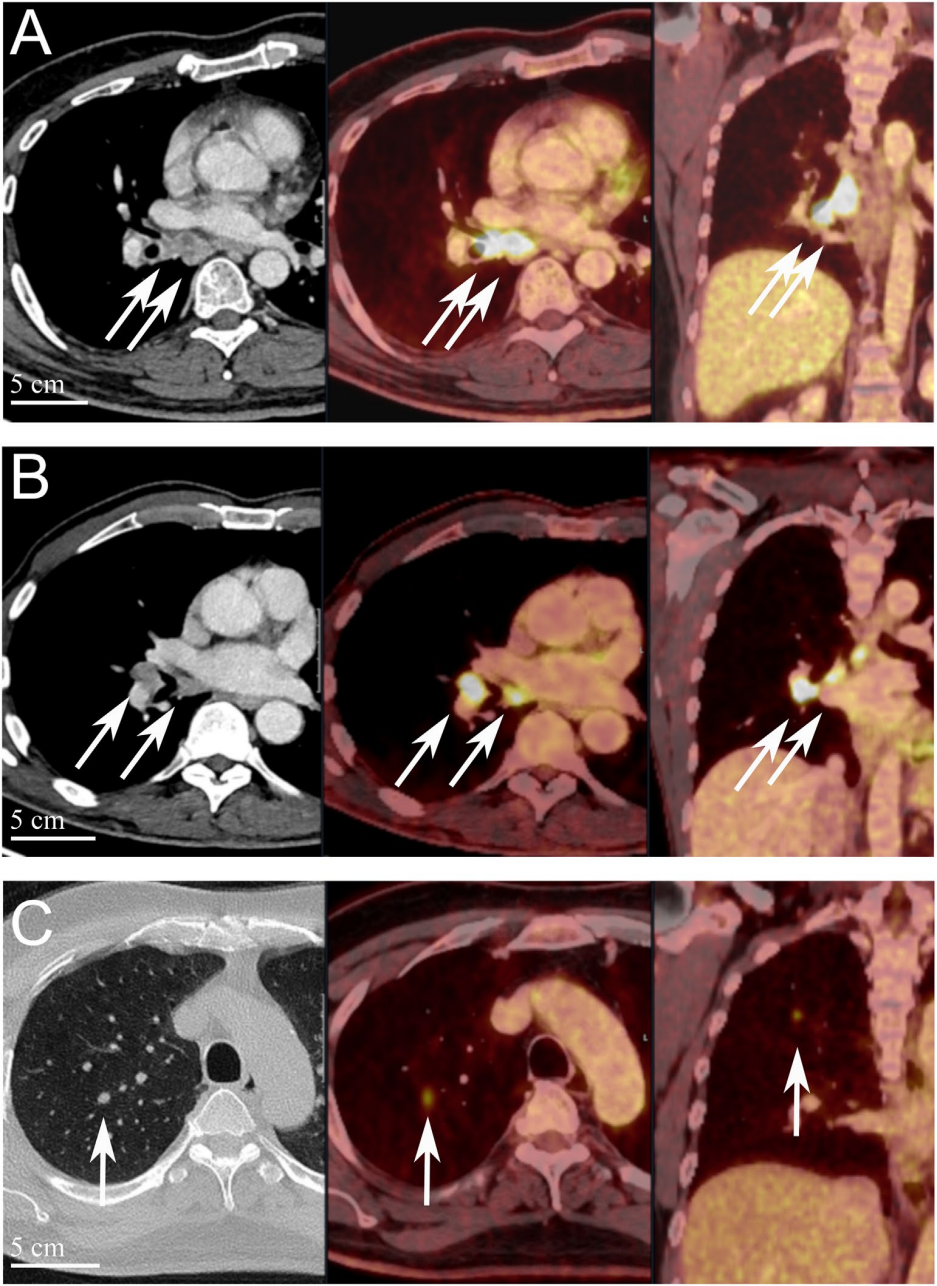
^18^F‐fluoro‐2‐deoxy‐D‐glucose positron emission tomography combined with computed tomography (FDG PET/CT) images of thoracic lesions in three different penile cancer patients. (A) FDG PET/CT positive lesion in right hilar lymph nodes (arrows) present 7 months after the patient was treated for an inguinal node‐positive penile cancer. (B) FDG PET/CT positive lesion in right hilar lymph nodes (arrows) present 14 months after the patient was treated for an inguinal node‐positive penile cancer. (C) Concurrent FDG PET/CT positive lesion in right upper lobe (arrows) of a patient that were simultaneously diagnosed and treated for an inguinal node‐positive penile cancer.
